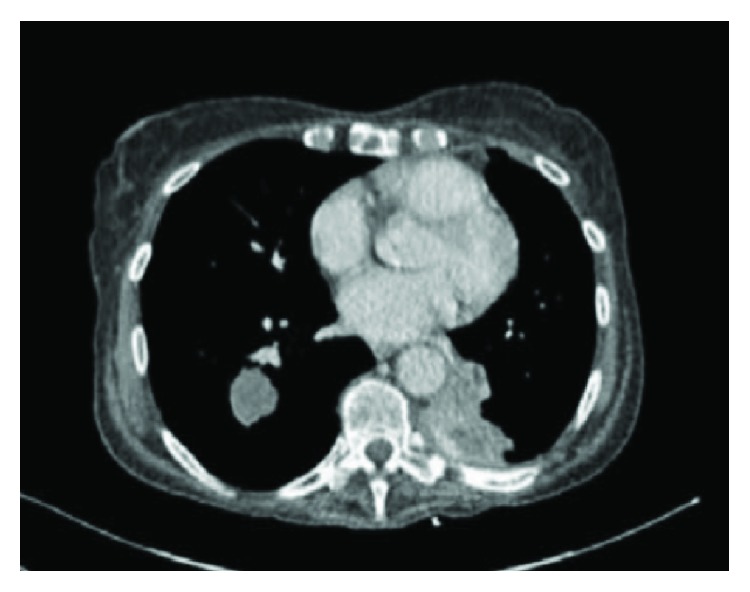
Restaging CT post 9 cycles of Nivolumab. There was a reduction of the RLL mass post 9 cycles of Nivolumab.
